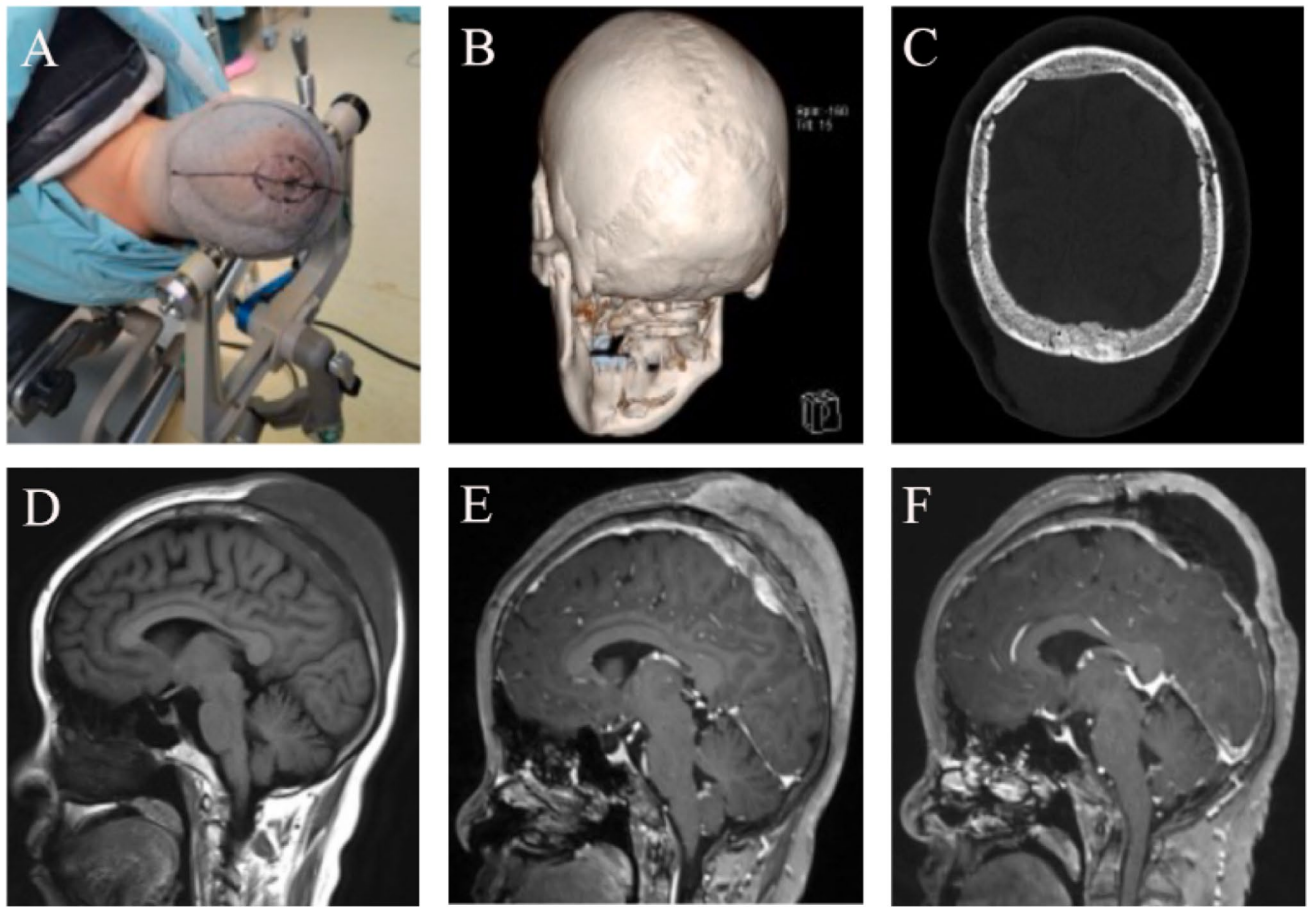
Imaging Findings Before and After Treatment in a 48-Year-Old Female with Scalp-Intracranial RDD. (A) Neuronavigation was used to mark the intracranial lesion site, and a wide-based flap was designed around the subcutaneous lesion. (B) Preoperative 3D CT showed no obvious erosion or destruction of the sagittal suture or the outer table of the skull. (C) Postoperative CT showed patchy high-density areas within the bone plate, suggestive of tumor-like changes in the bone. (D) Preoperative head MRI T1 sequence showed the bone plate appearing hypointense, with both intracranial and subcutaneous tumors displaying homogeneous low signal. (E) Preoperative enhanced MRI showed enhancement of the bone plate, intracranial tumor, and subcutaneous tumor, all presenting with high signal. (F) Postoperative follow-up enhanced MRI revealed subcutaneous fluid accumulation and a hypointense bone plate, suggesting ischemia due to the loss of blood supply in the bone flap.
